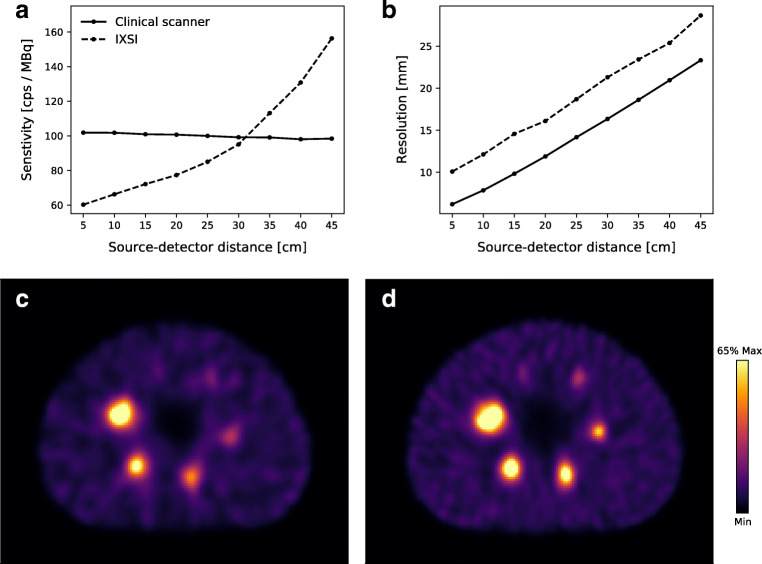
The nuclear image quality evaluation. a The detector sensitivity and (b) the spatial resolution, as a function of the distance from the source to the detector, for IXSI and one detector head of the clinical scanner. c The SPECT reconstruction from IXSI. d The SPECT reconstruction from the clinical scanner
